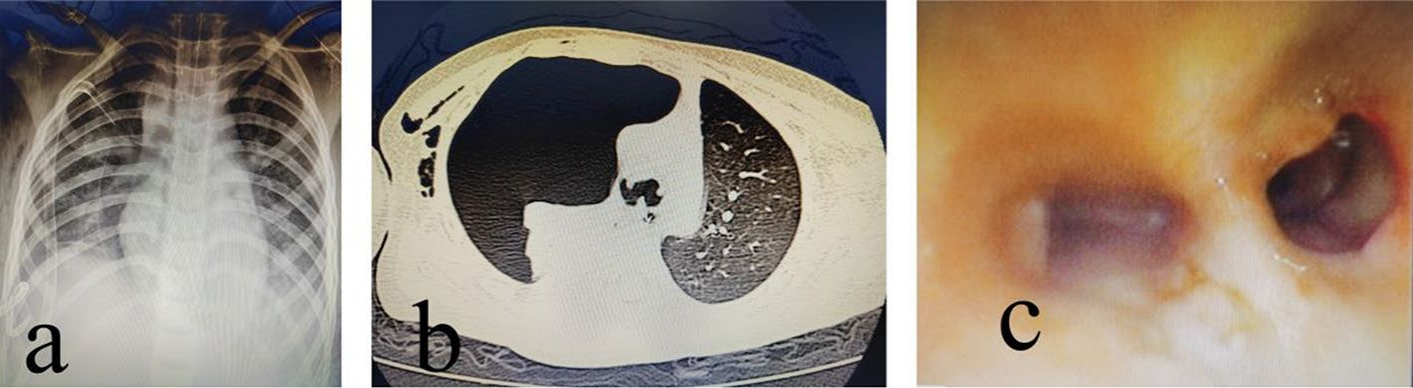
(a) Preoperative X-rays showed two drainage tubes inserted after treatment for right pneumothorax (b) Chest CT showed right pneumothorax (c) Bronchoscopic image of main bronchus rupture
